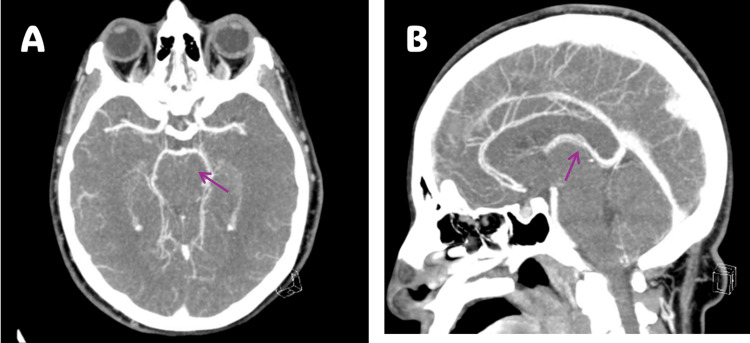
Computed tomography (CT) brain images. (A) Axial CT brain and (B) sagittal CT brain, both demonstrating no intracranial hemorrhage or venous sinus thrombosis, with findings suggestive of early diffuse cerebral edema (arrows).
